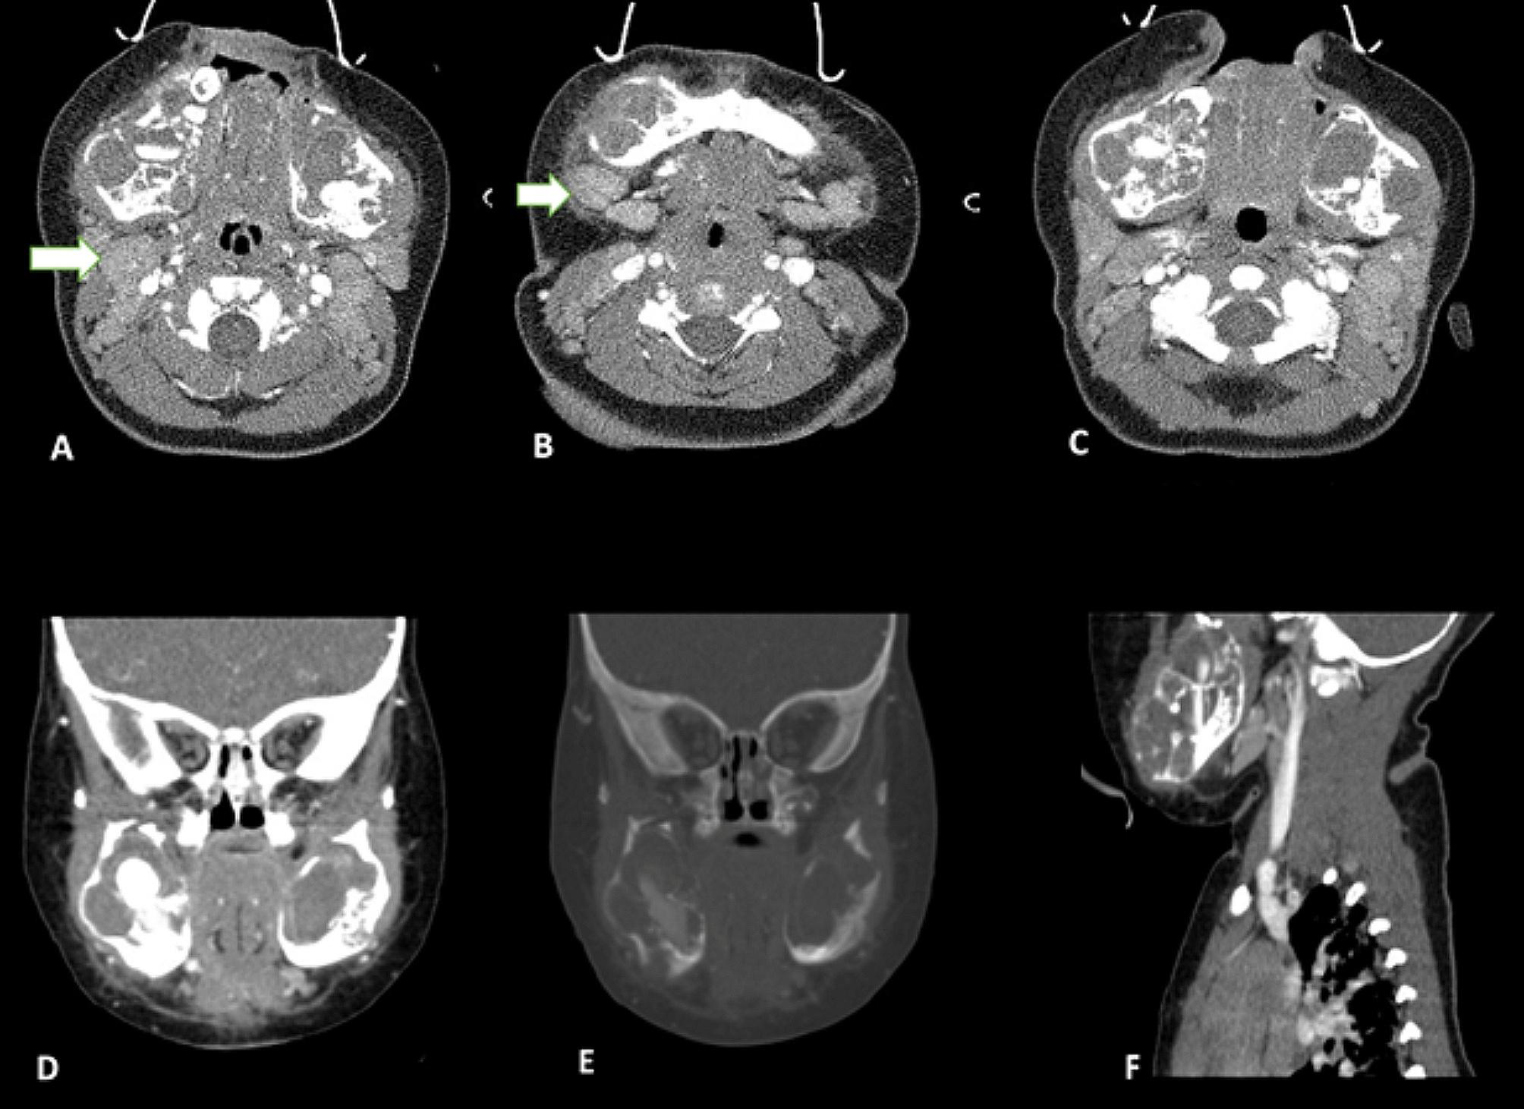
Axial (A, B, C), coronal, bone window (D, E), and sagittal F) images of enhanced CT scan of the face with 3D reconstruction and Intravenous contrast. The images reveal an expansile multi-loculated lytic mass in the body of both mandibular bones without periosteal reaction or soft tissue component, measuring about 37 × 29 × 43 mm on the right side and 34 × 24 × 37 mm on the left side. Both maxillary bones appear normal. Multiple lymph nodes are seen in the submandibular and both jugular chains (white arrow); the largest on the right side measures 15 × 9 mm, and that on the left measures 11 × 7 mm. An enlarged lymph node measuring 15 × 9 mm in the anterior aspect of the trachea
